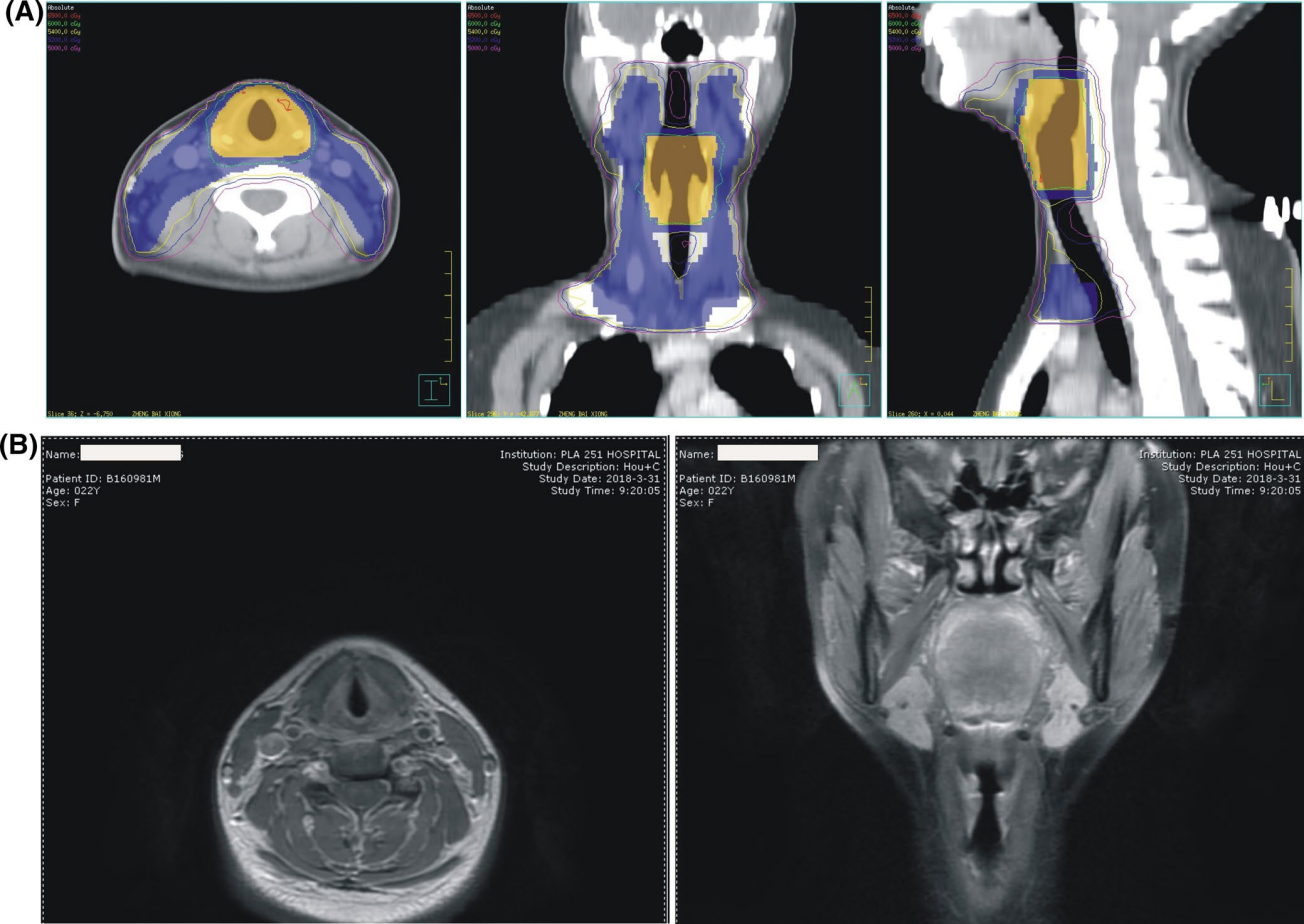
(A) Program of intensity‐modulated radiation therapy; (B) Neck magnetic resonance imaging examination 15 mo after vocal cord resected
